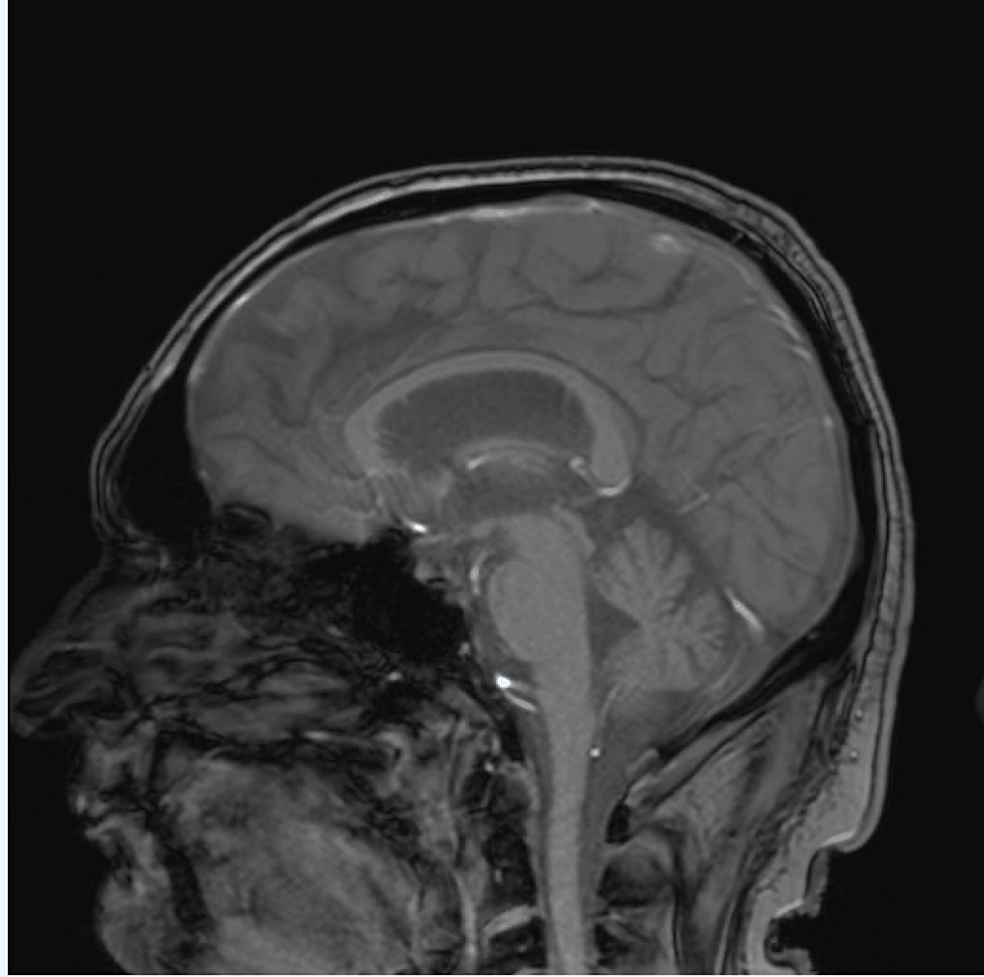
Brain MRI Sagittal view showing the cerebrum and cerebellum. Mild global parenchymal volume loss is noted.
